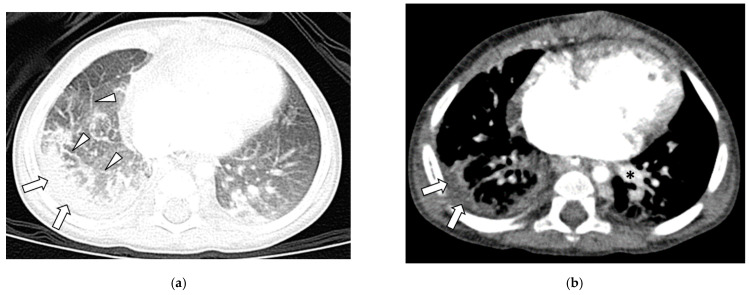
Fourteen-month-old girl with right sided pulmonary vein stenosis. (a): Axial lung window CT image demonstrates septal thickening (arrowheads) and pleural thickening (arrows) in the right hemithorax. (b): Axial contrast-enhanced soft tissue window CT image shows pleural thickening (arrows) in the right hemithorax. Right pulmonary veins are absent. Left pulmonary vein (asterisk) is visualized.
